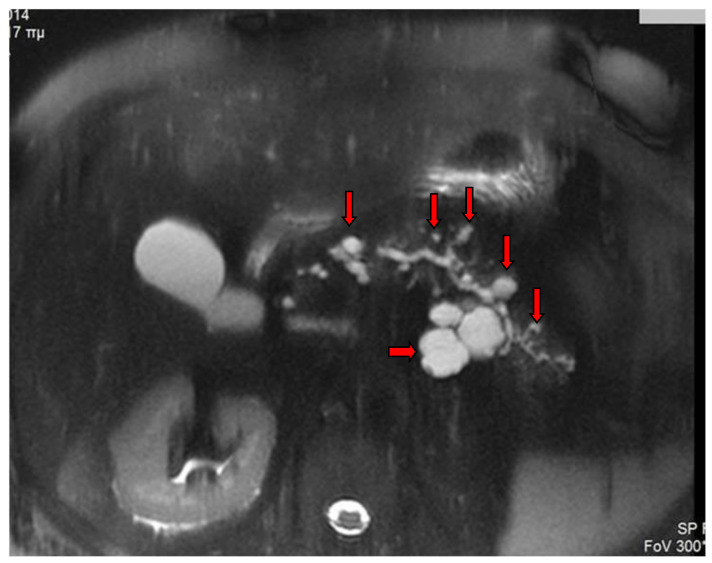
T2-w fs MR image shows many IPMNs of different sizes and morphology throughout the pancreas (arrows).
